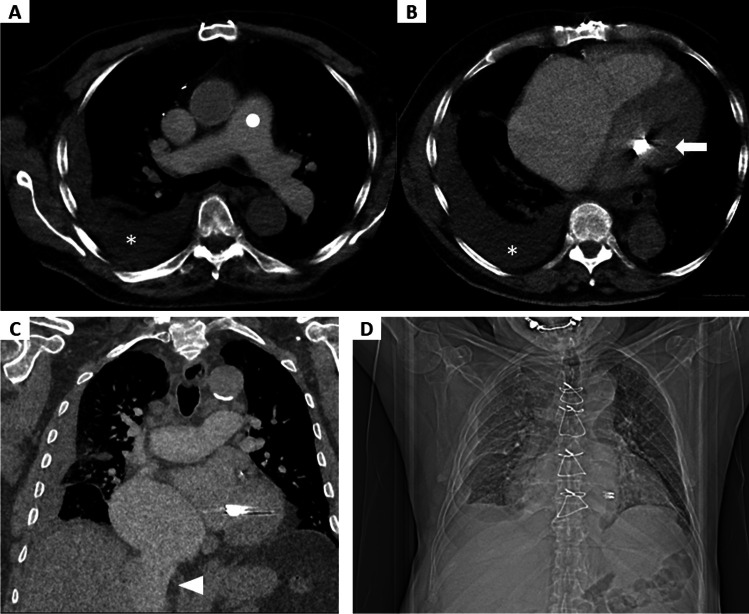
Example of an examination with adequate evaluated subjective image quality and several artifacts. Example of a high-pitch CTPA examination with adequate subjective image quality (rating score 2) without pulmonary arterial emboli in a 90-year-old male patient (BMI 24.9 kg/m^2^) (A–C). Scout-view of the same patient during the CT examination (D). The patient had severe heart co-morbidities, e.g., aortic valve replacement, 2 × mitral clipping because of mitral regurgitation, pulmonary hypertension with right-sided atrial dilatation and ride-sided pleural effusion (*) (A, B). Axial view with a circular region of interest (circle) and measured contrast opacification of 130.4 ± 28.4 HU (A). The arrow indicates radial beam-hardening caused by the mitral clips (B). Retrograde opacification of the inferior vena cava and hepatic veins (arrowhead) (C). The scout-view revealed signs of a severe heart disease. This should lead to a critical evaluation of the chosen protocol (D). BMI, body mass index; CTPA, computed tomography pulmonary angiography; HU Hounsfield units
